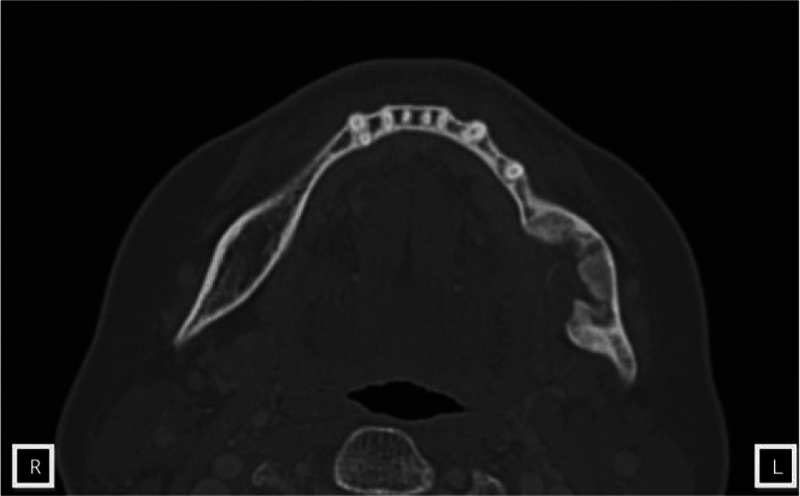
The tumor of the left mandibular angle in CT scan. Irregular loss in the bone structure sized 30 × 37 × 20 mm. CT = computed tomography.
